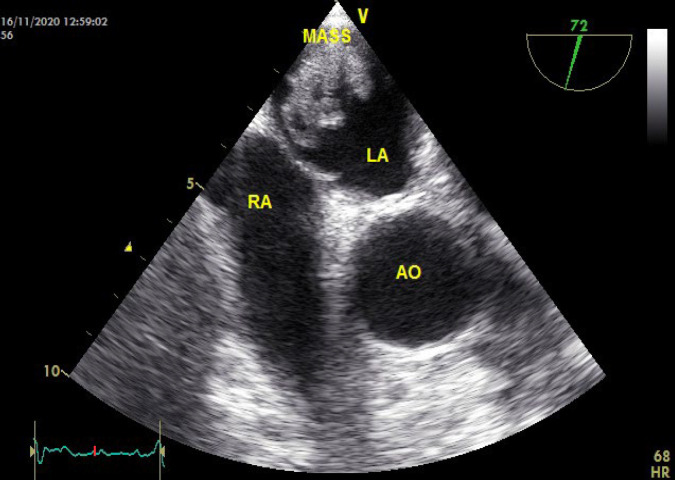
A large size mass (4×2.5cm) in the left atrium protruding into the left upper pulmonary vein shown in the short axis transesophageal echocardiogram view
